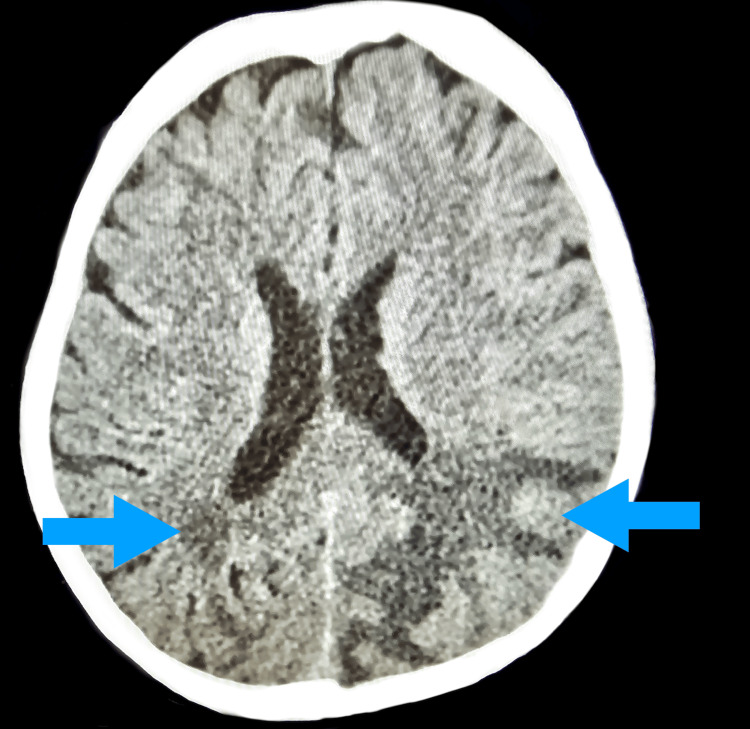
Axial brain CT demonstrating bilateral occipital hypodensities in the posterior white matter (blue arrows), consistent with vasogenic edema.
